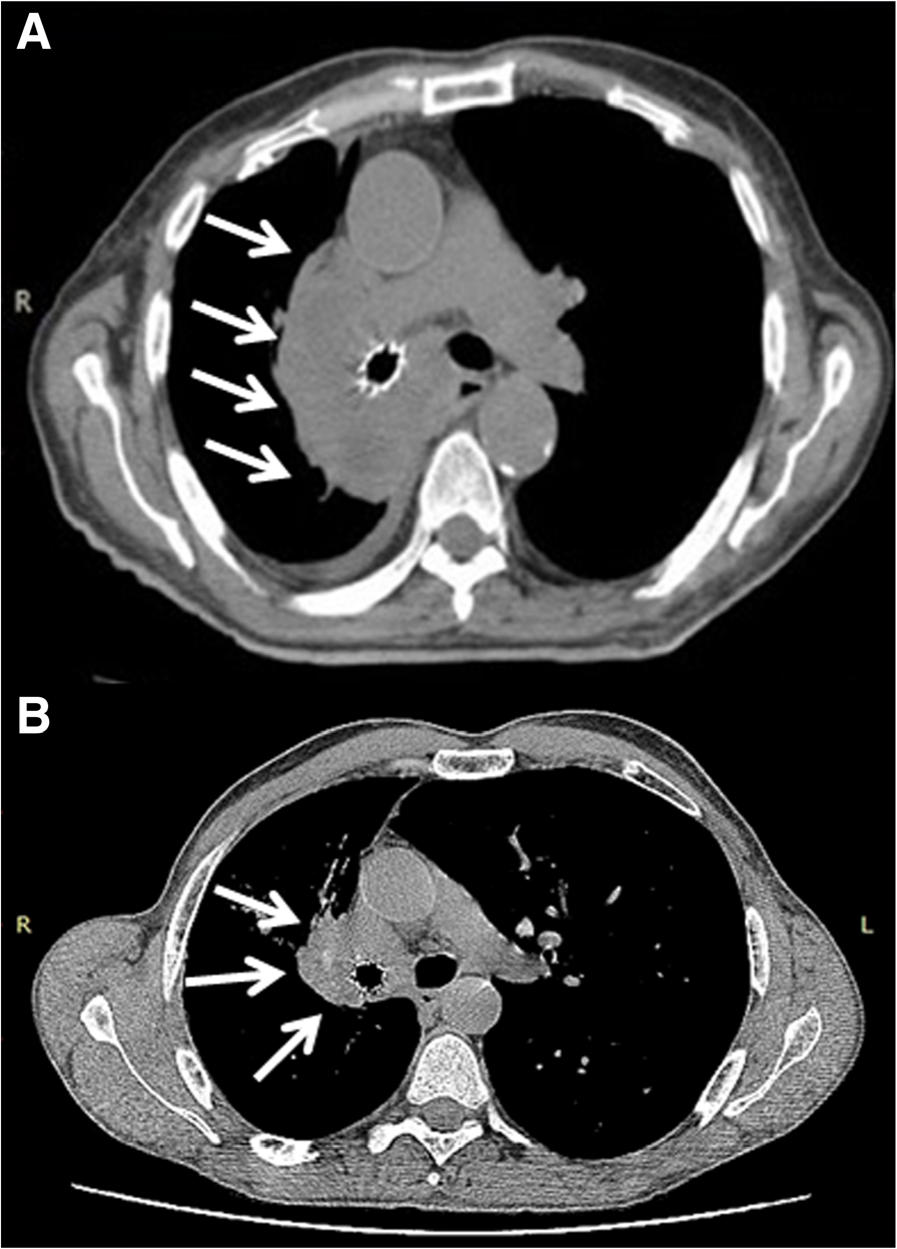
Chest CT scan performed before (Part a), and 16 weeks after immunotherapy (Part b) showed a reduction in tumor size (white arrow) without sign of lung infection
